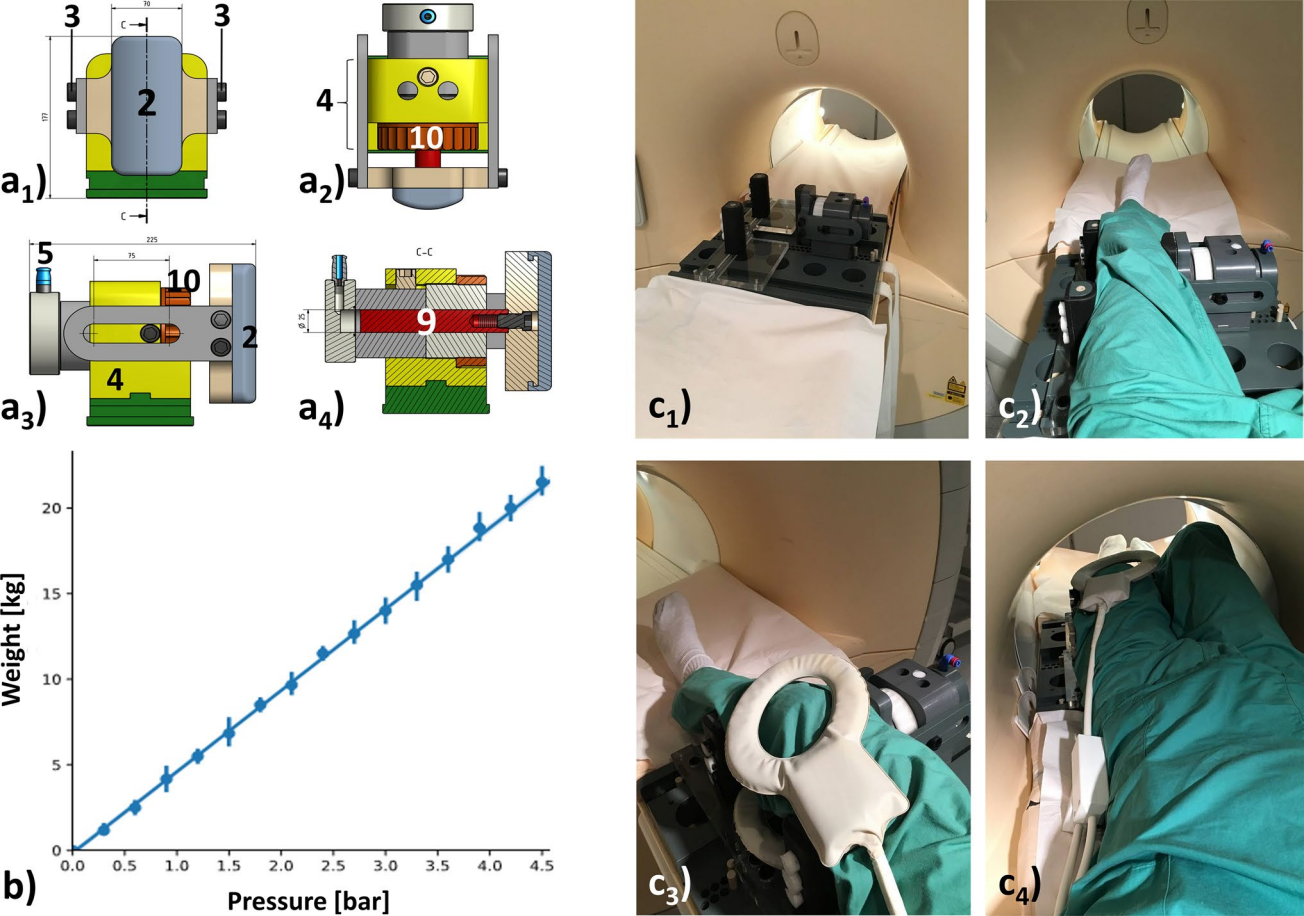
CAD schemes of the pneumatics (a), pressure vs. weight calibration curve (b), and operation of the MRI-compatible varus–valgus loading device in a 3.0-T MRI scanner (Achieva, Philips) (c). a Front view (a_1_), top view (a_2_), side view (a_3_), and central cross-section (a_4_) of the pneumatics. The loading piston (9) has a diameter of 25 mm (= 2.5 cm) and may be adjusted in its horizontal position by means of a coarse set screw (10). Otherwise, component numbering and color coding as in Fig. 1. Units of dimensions are (mm). b Theoretical (solid line) and measured (data points) associations between set pressure levels (*x*-axis) and resultant weights or forces (*y*-axis) are plotted. Points give means, while bars give standard deviations (of three measurements). c Stepwise loading for eventual MR imaging, i.e. device in the unloaded configuration positioned on the patient bed (c_1_) and loaded with the senior author’s left lower extremity for varus loading (c_2_). Multi-purpose measurement coils were used for MR imaging and positioned above and below the knee joint (c_3_). Final measurement position in the scanner with the contralateral lower extremity placed on the pneumatics (c_4_)
